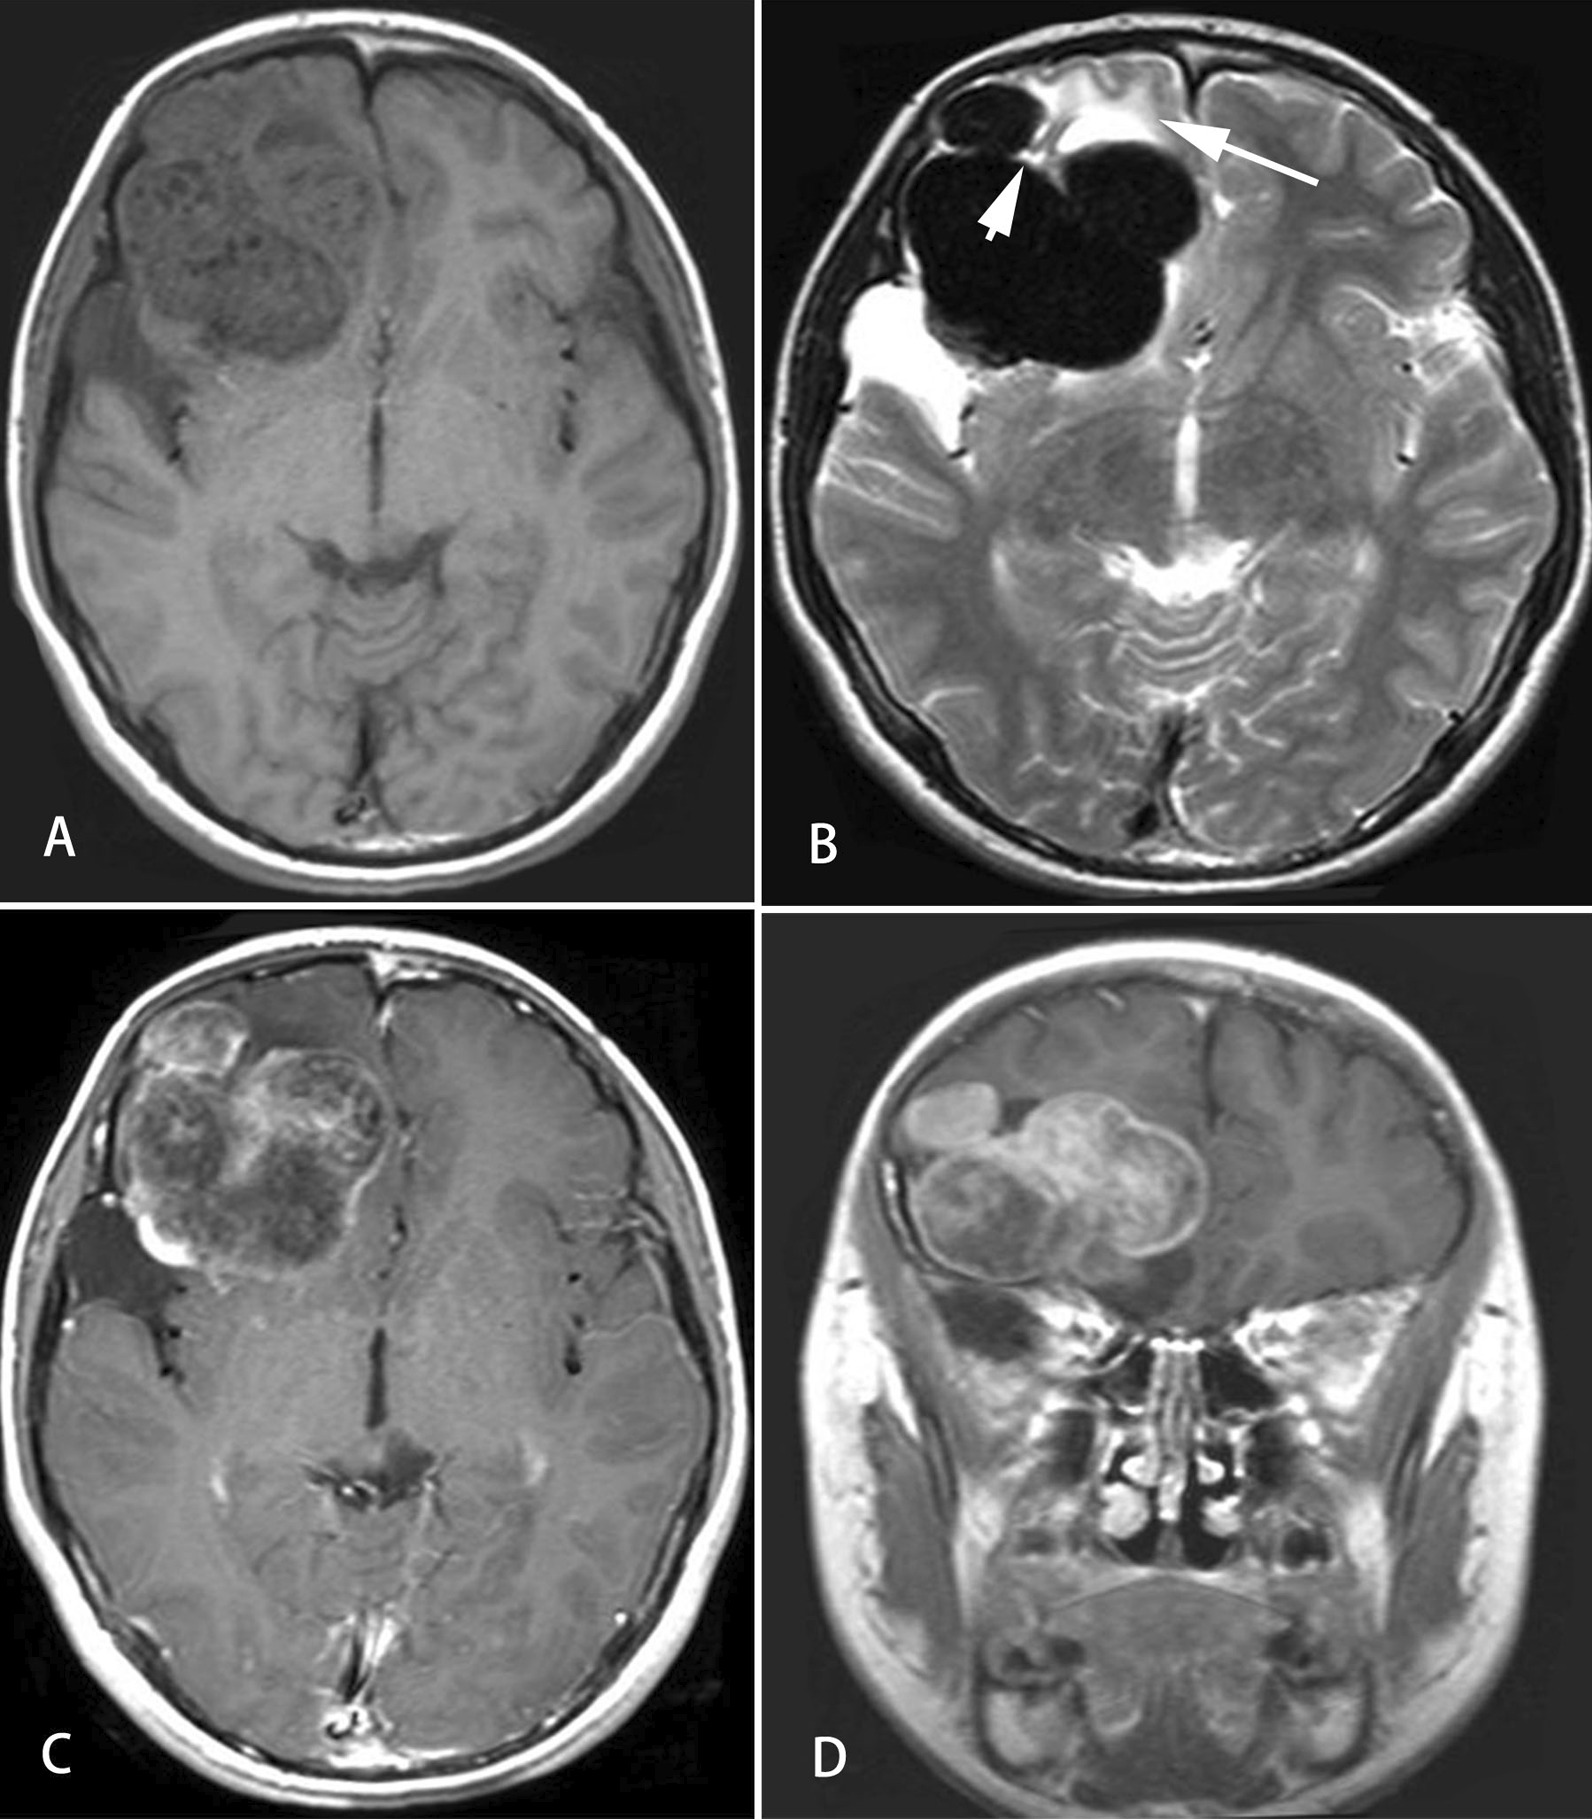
MRI findings of a 12-year-old man with intraparenchymal schwannoma. A well-defined heterogeneous mass with peritumoral edema (arrow) and cystic degeneration (arrow head) is observed in the right frontal lobe (A and B). The mass shows hypo-intensity on T1 WI and hypo-/hyper-intensity on T2WI. The hypo-intensity area on T1WI and T2WI was suggestive of calcification (B). Heterogeneous, marked and delayed enhancement could be observed on the axial and coronal T1WI (C and D)
